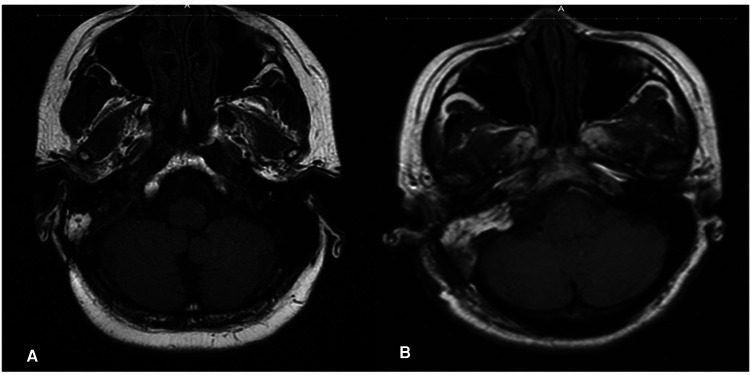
MRI T1 showing the fat packing in the patient with no stenosis (A) and stenosis (B) in the early postoperative period.
